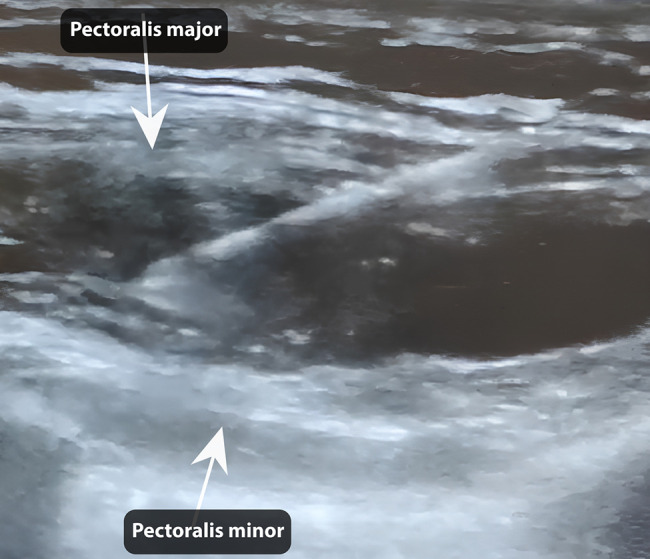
An ultrasound image showing the pectoralis major and minor muscles divided by anesthetic.
